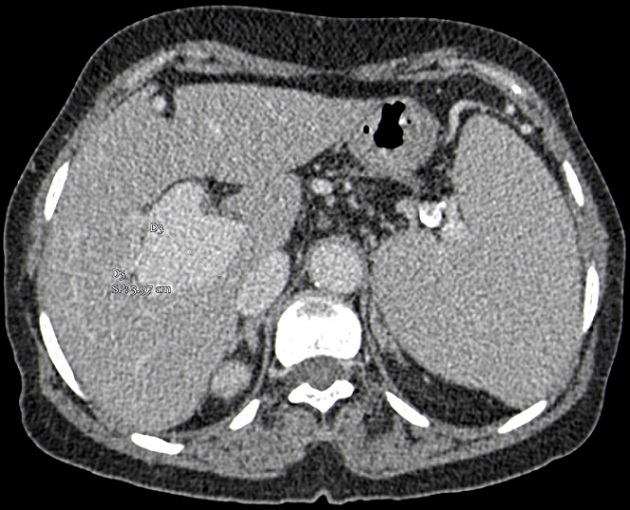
Axial reconstruction of a portal-phase angio-CT showing portal aneurysm located in the portal bifurcation
